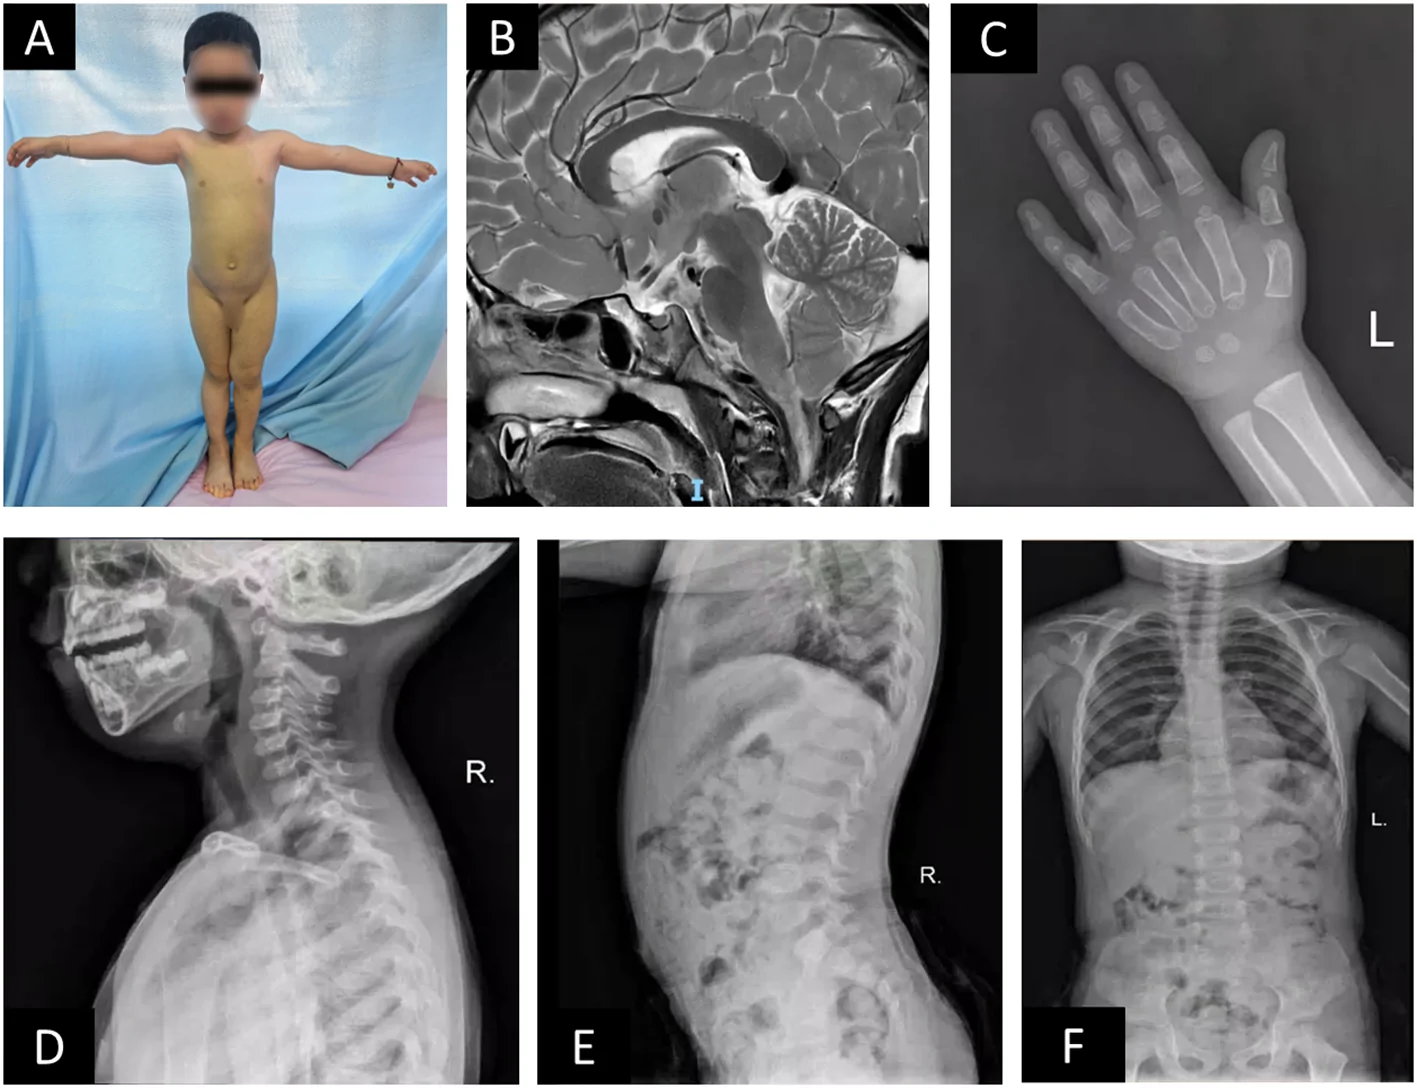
Clinical and radiographic features of the proband. (A)Photograph demonstrating proportionate short stature. (B) Pituitary magnetic resonance imaging (MRI) showing no structural abnormalities. (C) Bone age assessment consistent with chronological age. (D–F) Full-spine radiographs showing mild scoliosis without definitive vertebral malformations.
